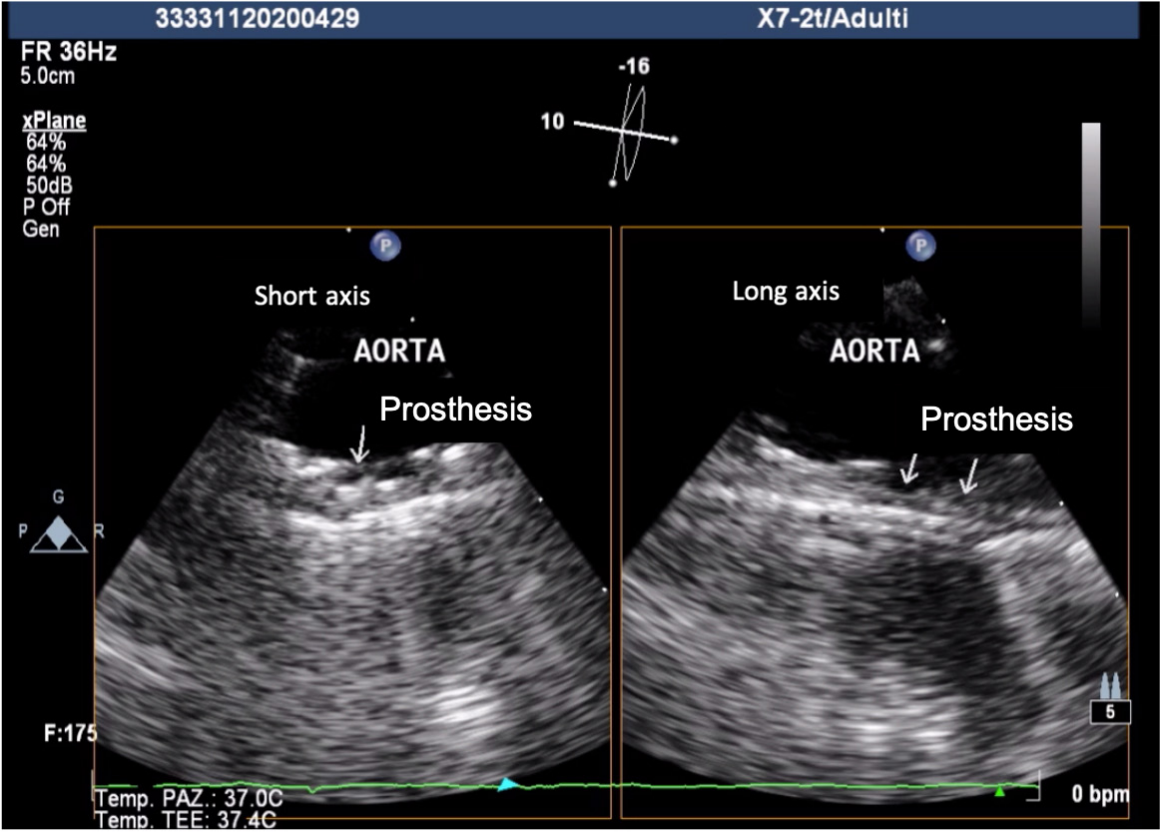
Intraoperative transesophageal echocardiography showing complete exclusion of aortic mural thrombus after endograft deployment. Short axis (left) and long axis (right).
